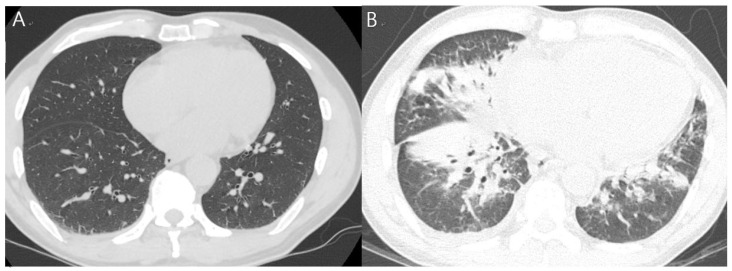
Chest computed tomography (CT). (A) Chest CT on admission demonstrating probably dependent atelectasis of the basal aspect of the right lower lobe and (B) on day 6 after admission indicating new-onset extensive consolidation and ground-glass opacity of the right middle lobe, lingular segment, and both lower lobes with increased right pleural effusion.
